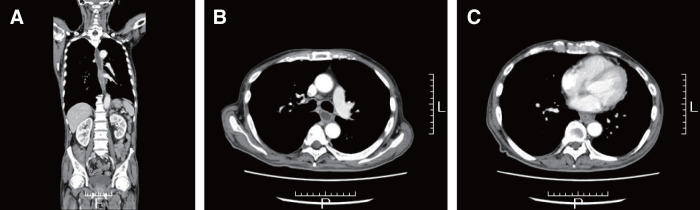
CT findings. Circumferential stenosis was observed in the same segment as the endoscopic findings. (A) Sagittal view. (B) Dilatation of the proximal esophagus was noted. (C) Circumferential stenosis.
